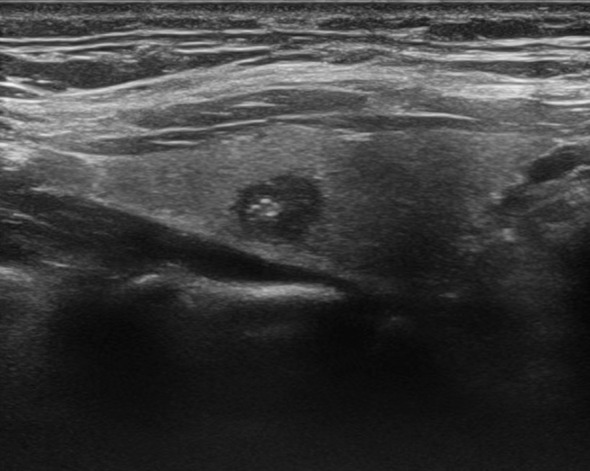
Representative examples of raw ultrasound images.
